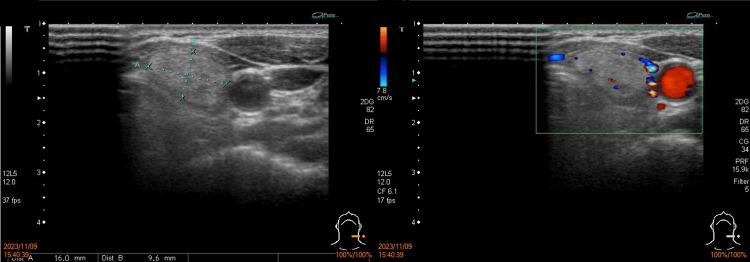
Sonogram of the left thyroid nodule A well-encapsulated and hyper-isoechoic solid lesion with a smooth margin, measuring 16 x 9.6 mm, was detected in the left thyroid gland under sonogram. It was wider than tall and showed hypovascularity under Doppler sonography, and was characterized as EU-TIRADS 2. EU-TIRADS: European Thyroid Imaging Reporting and Data System
